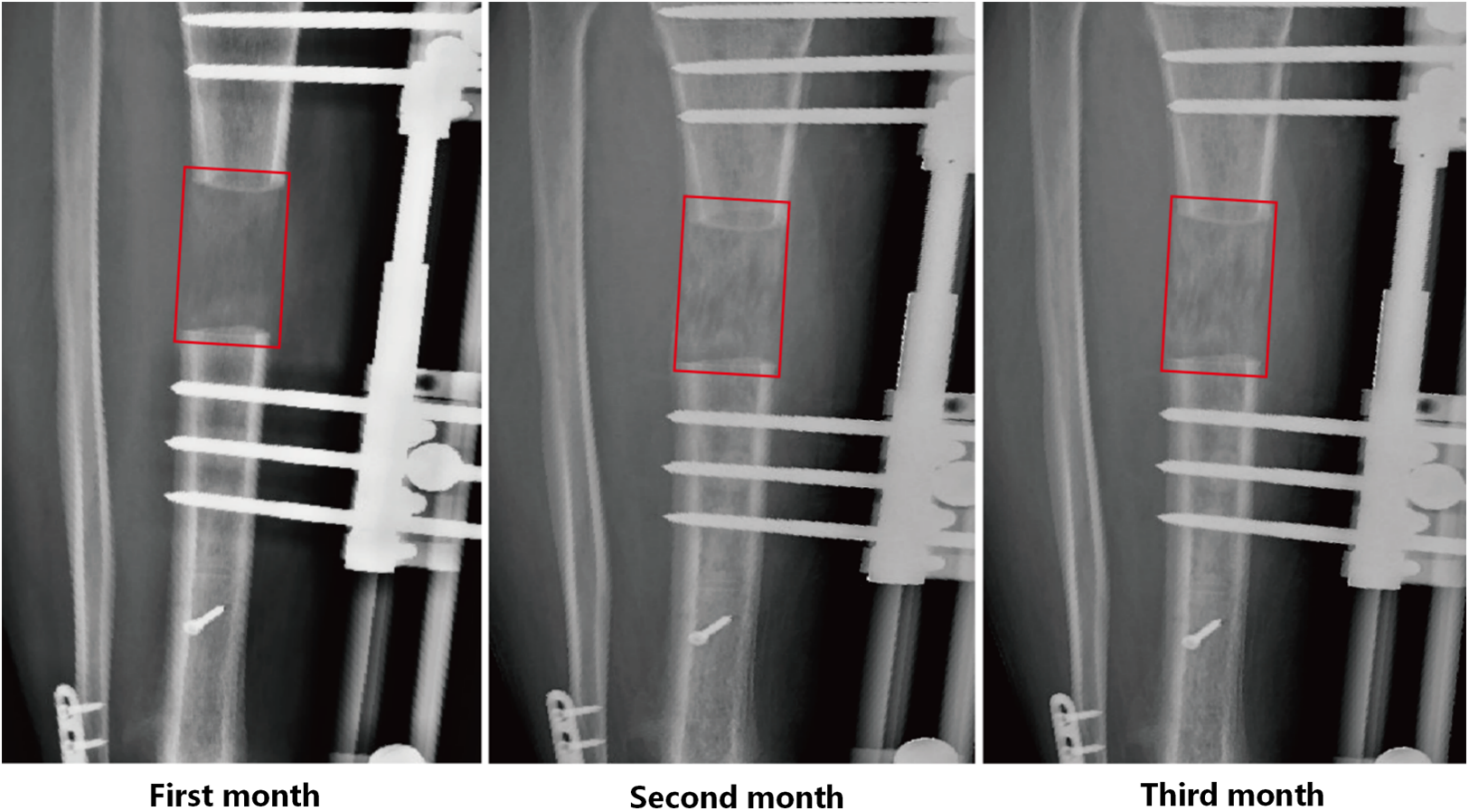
Radiographic assessment of bone regeneration during bone transport.
